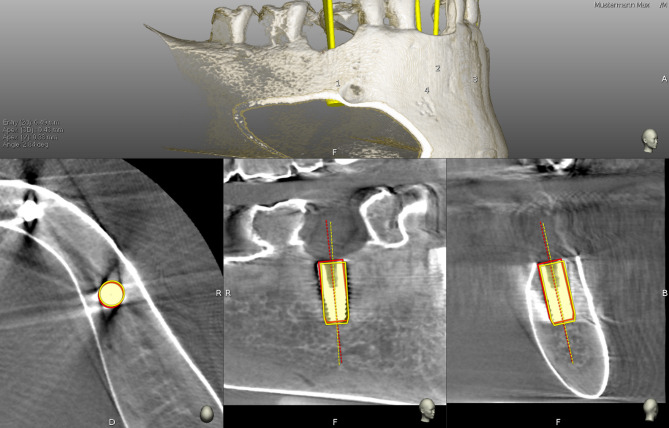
Superimposition of pre- and postoperative CBCT datasets. Abbreviations: CBCT (cone-beam computed tomography); grey shades: CBCT data; yellow: planned implant position, red: transferred implant position, top left: calculated global deviations
